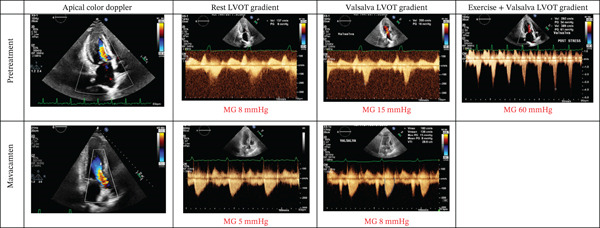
Transthoracic echocardiographic images demonstrating increased LVOT gradient with both exercise and Valsalva maneuver and incremental improvement after treatment with mavacamten.
